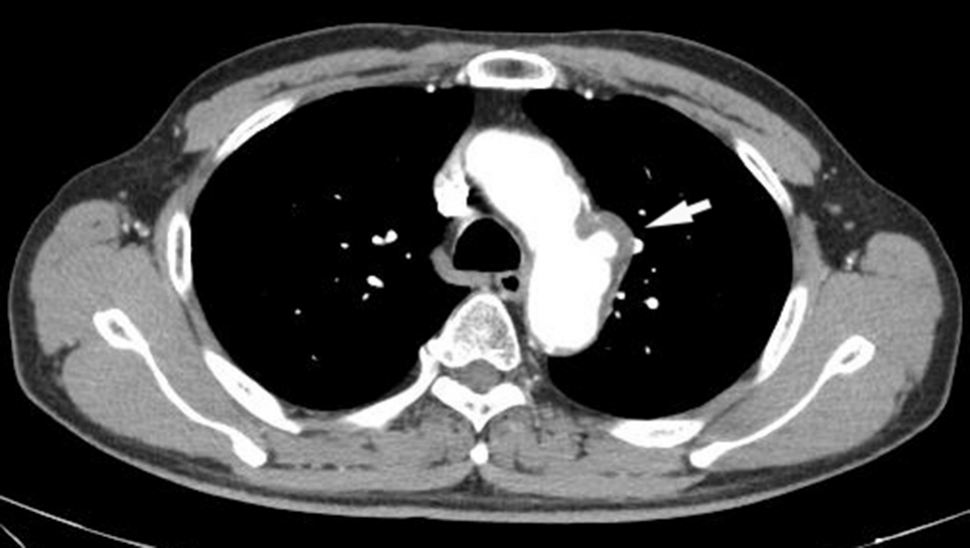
Enhanced computed tomography demonstrated a saccular aneurysm in the aortic arch (arrow)
